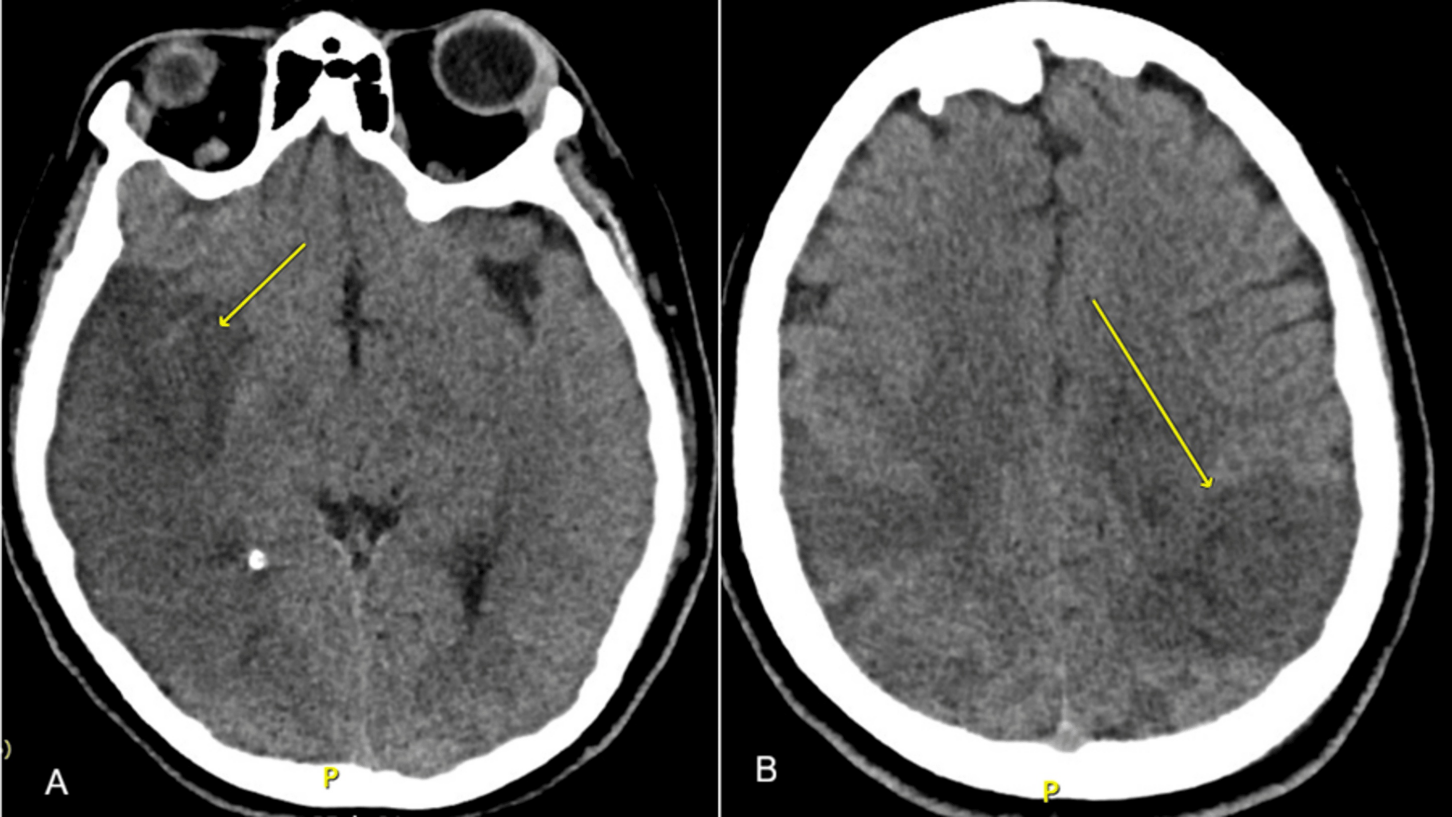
Brain computed tomography (CT) (A) Non-contrast axial cranial CT showing ischemic changes in the right parietotemporal region (arrow). (B) Ischemic area in the left parietal lobe (arrow), associated with cerebral edema.
